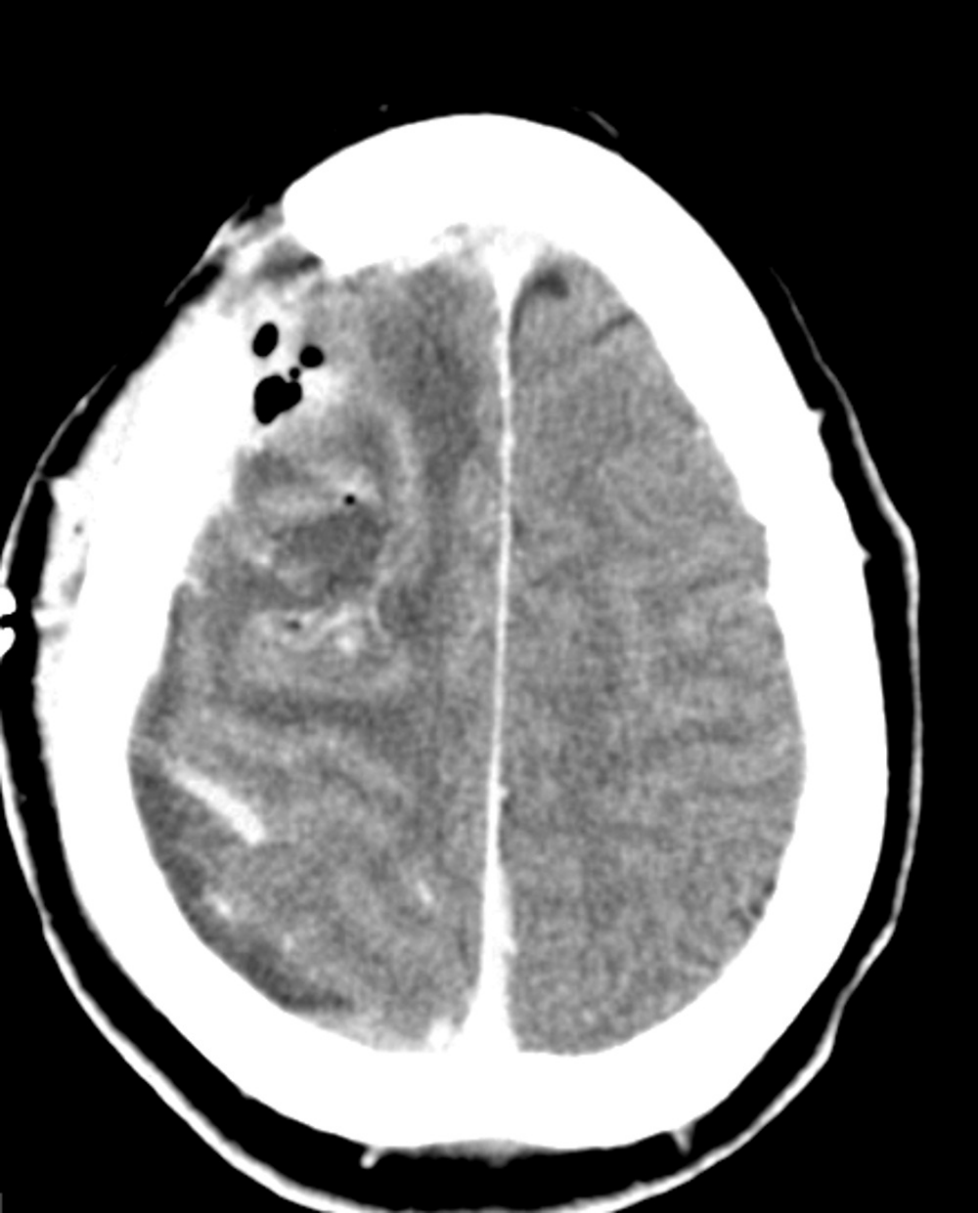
Postoperative CT head with contrast after abscess evacuation
